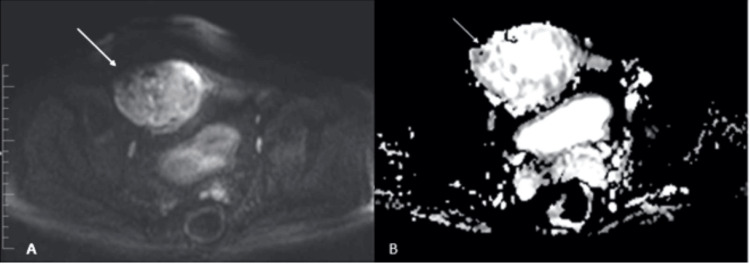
Axial diffusion (A) and ADC (B) magnetic resonance imaging showing patchy restricted diffusion also noted in the periphery of the non-necrotic region (white arrows) ADC: apparent diffusion coefficient
